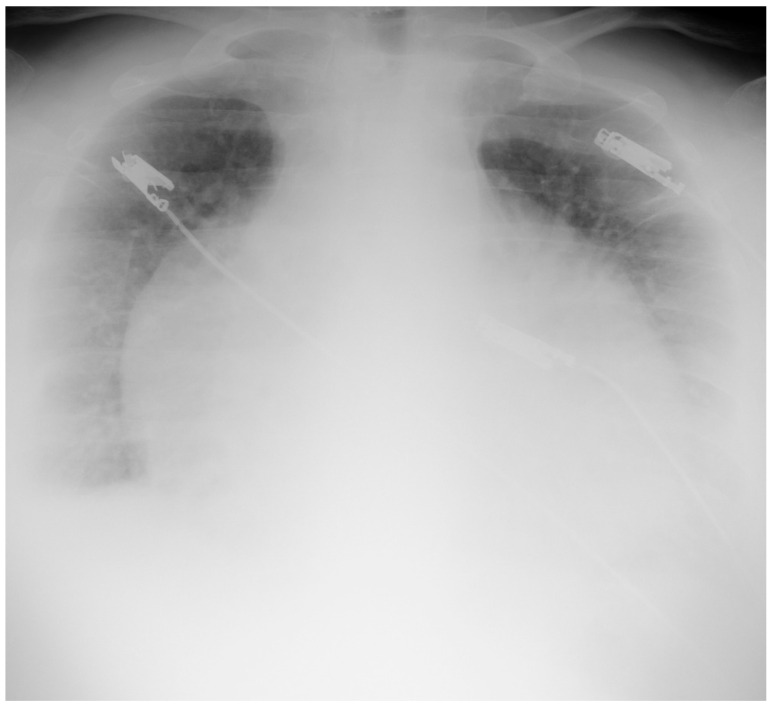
Chest radiograph in supine position shows significantly enlarged cardiac silhouette, signs of pulmonary congestion, and increased homogeneous density superimposed over the lungs due to bilateral pleural effusion.
